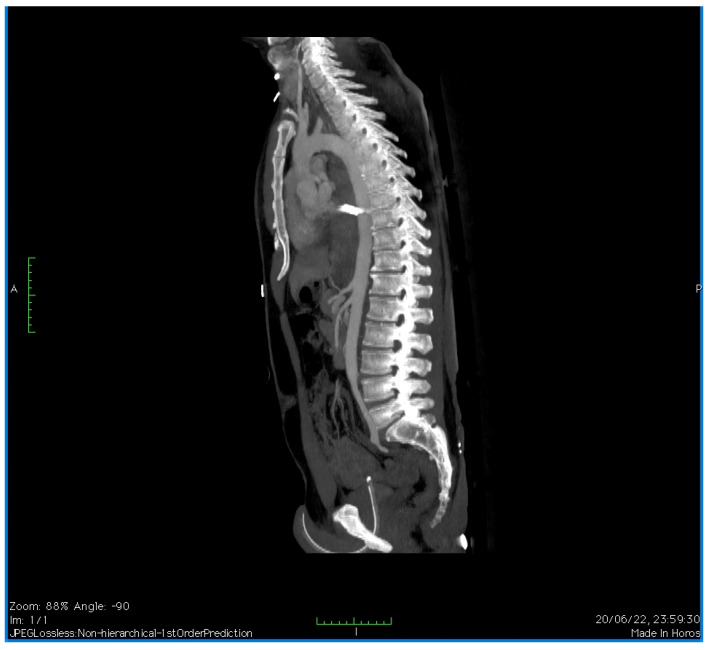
CTA of the chest revealing penetrating thoracic aorta injury (sagittal view).
